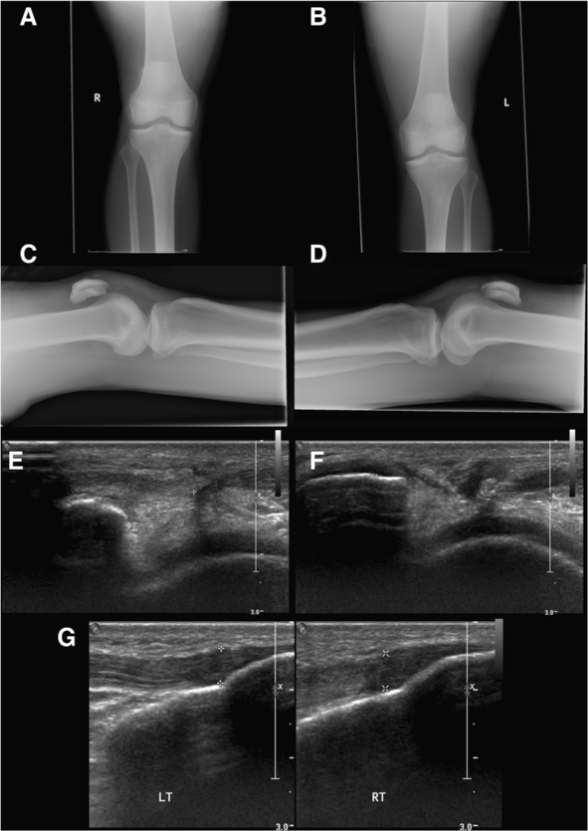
Pre-operative imaging demonstrating bilateral patellar tendon rupture: Xray imaging (a) Anteroposterior (AP) view of the right knee, (b) AP view of the Left knee. c Lateral view right knee and (d) lateral view left knee. These four images indicate bilateral patella alta. Ultrasound imaging is shown in: (e) Saggital view of the right patellar tendon demonstrating a 1.65 cm defect indicating rupture, (f) Saggital view of the left patellar tendon demonstrating a 1.49 cm retraction indicating rupture. g Bilateral views of the distal tibial insertions indicating that this was intact bilaterally
